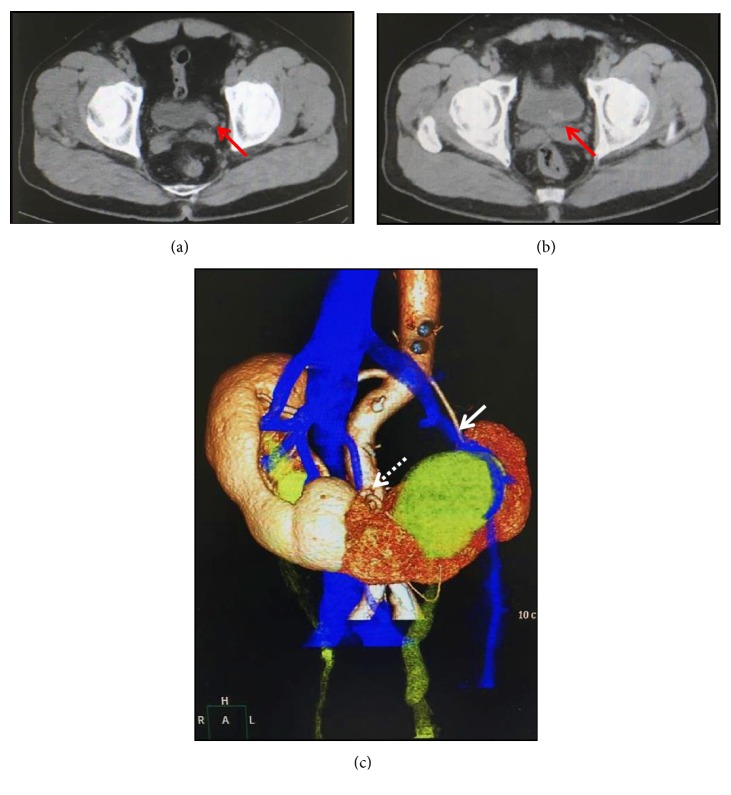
(a), (b) Pelvic CT images showing a left lower ureteral tumor near the ureteral orifice (red arrows). (c) Three-D CT imaging. The left renal artery (white arrow) and an aberrant renal artery (white dotted arrow) supplied the left part of the horseshoe kidney.
